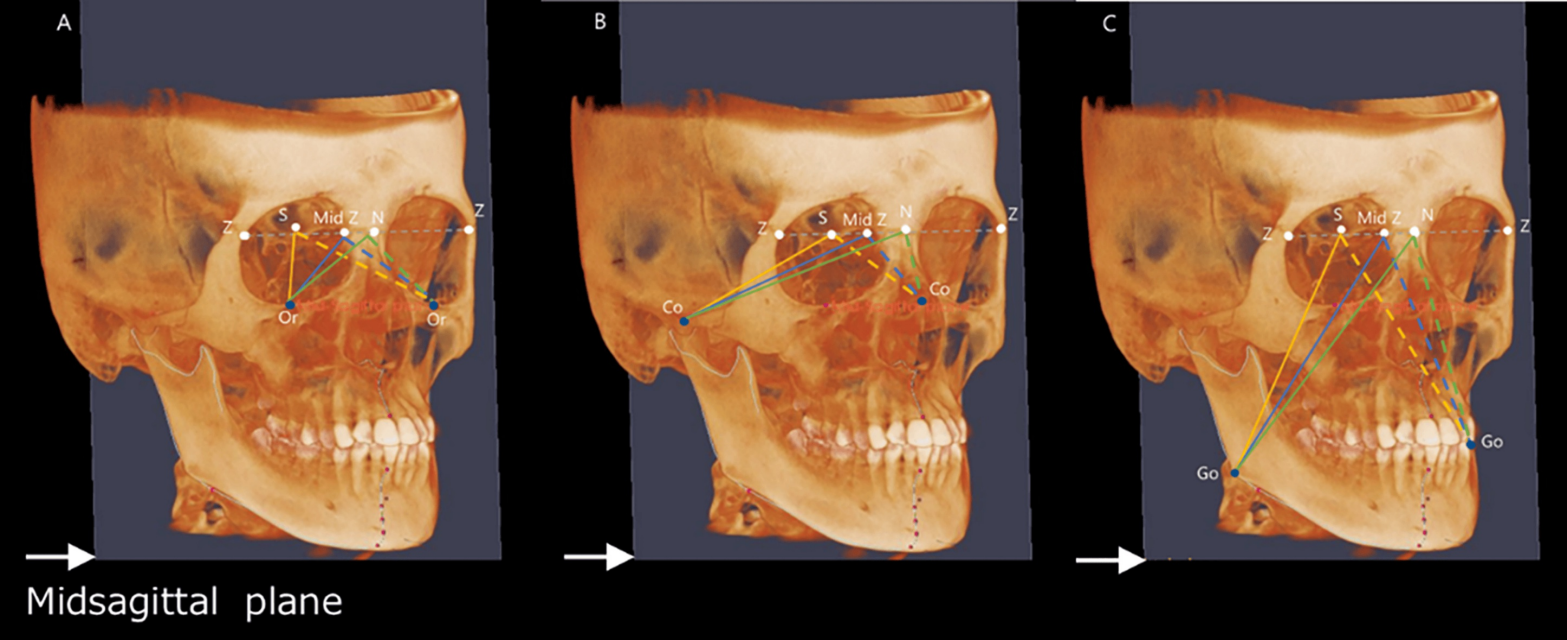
Evaluation of each facial asymmetry index using cone-beam computed tomography images. A: distance measurement from each reference point to Or; B: distance measurement from each reference point to Co; C: distance measurement from each reference point to Go. Right side: solid line; left side: dotted line; S (sella): center of the pituitary fossa; N (nasion): nasofrontal suture at midline; Mid Z (midpoint of Z): midpoint of the line between both Z points; Z point: the most inferior point on the zygomaticomaxillary suture; Or (orbitale): the lowest points of the orbital rim; Co (condylion): the most superior point of the condyles;
